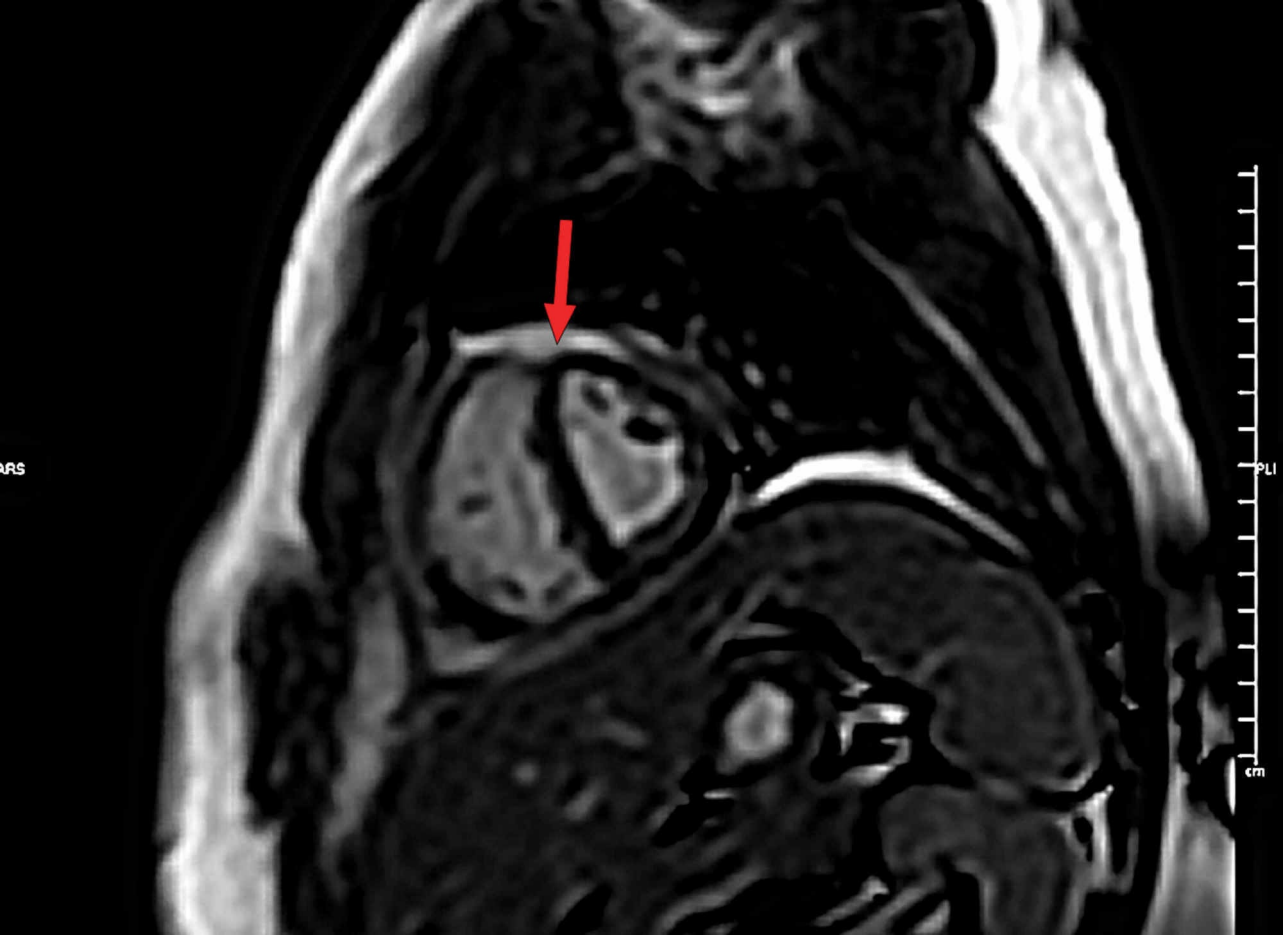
MRI short-axis view. Red arrow: septal bounce.
